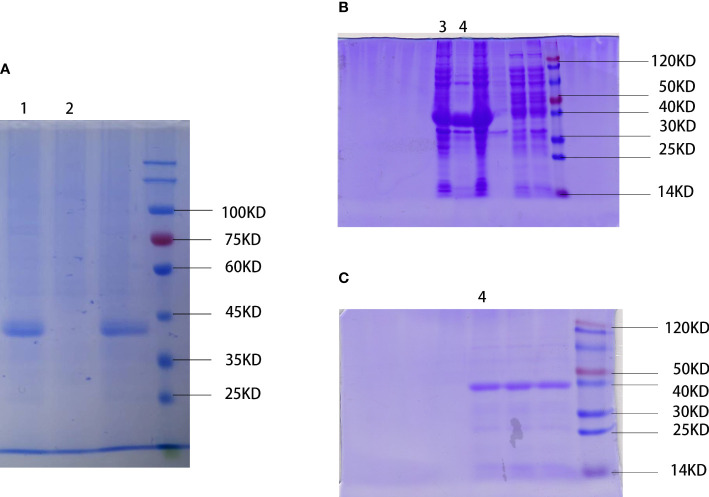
Extraction and preparation of water stress protein. (A) Physical extraction of water stress protein using pyruvate precipitation method. WSP is located near the 45 kD of protein marker. 1: WSP of the *“Nostoc commune” Vauch* after ammonium sulfate treatment. 2: crude extract. (B) Recombinant water stress protein created by genetic engineering. WSP is located near the 40 kD of protein marker detected by SDS-PAGE. 3: bacteriophage after ultrasonication lysis treatment. 4: WSP after affinity purification. (C) Purified water stress protein through nickel column purification (nickel purified), and anion exchange chromatography (anion exchange). 4: WSP after affinity purification.
